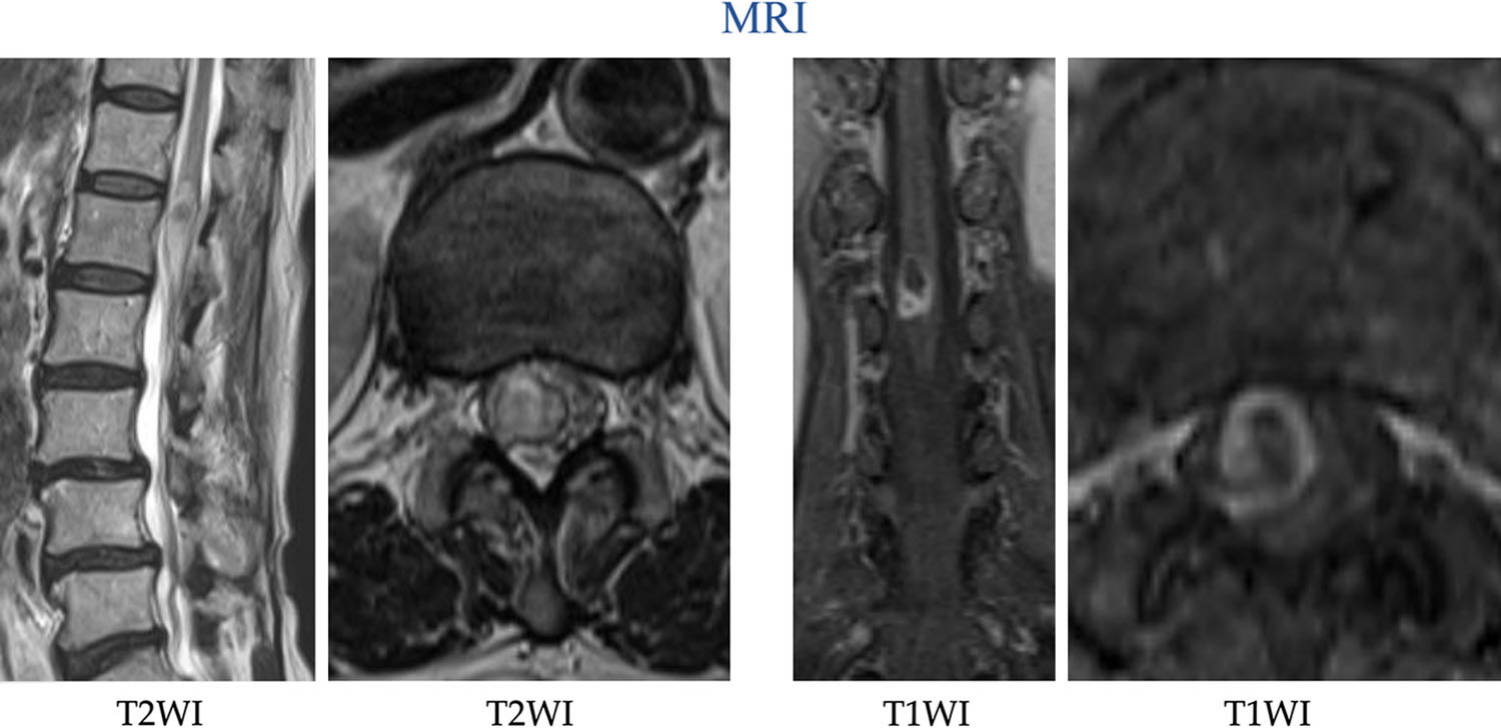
Left* T2-weighted MRI. Slight spondylolysis at both L3 and L4 was found; however, there was no lumbar spinal canal stenosis. At the T12/L1/L2 level, a spindle-shaped intradural lesion was revealed on a sagittal T2-weighted image. The entire outer area of the lesion was high-intensity, and a round inner area was low-intensity. On an axial T2-weighted image, the lesion was seen at the right side within the dura mater, causing the nerve filaments to shift to the left. The margin of the lesion was irregular and of low intensity, while the inner area was mixed high- and iso-intensity. *Right* enhanced T1-weighted MRI. Only the margin of the lesion was enhanced
